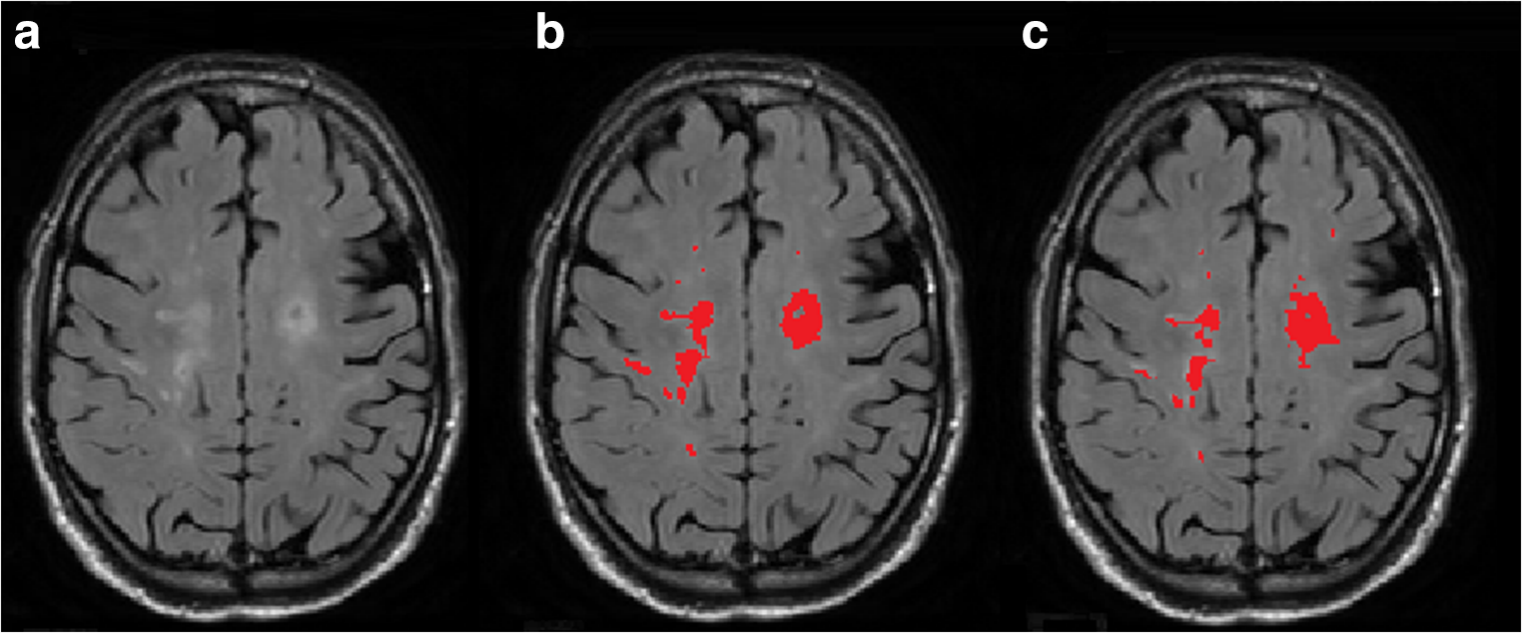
An example of lesion segmentation affected by FFR processing showing 2D FLAIR image (a), 2D FLAIR image with the lesion segmentation shown in red (8.30 mL) on full image (b), and 2D FLAIR image with the lesion segmentation shown in red (15.91 mL) on FFR-processed image with Defacing (c). Dice’s similarity index between the complete 3D segmentations obtained from the full image and FFR-processed image was 0.48
